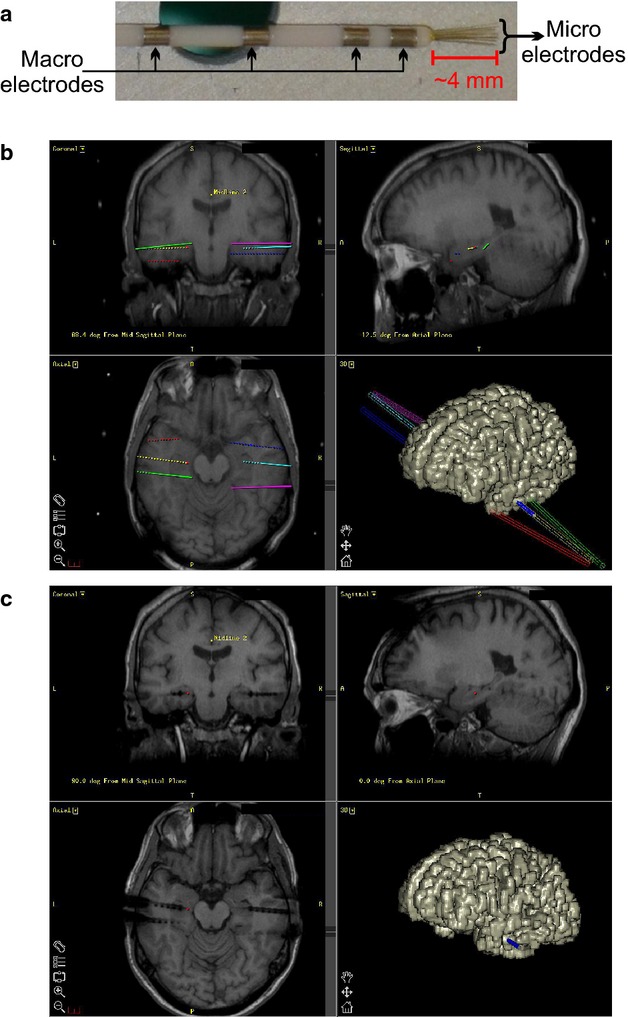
Surgical planning. (A) Before implantation, the microwires are inserted in the lumen of the polyurethane probe so they can be trimmed to the desired length (∼4 mm). (B) Based on a pre-operative MRI scan, computer software provides the stereotactic coordinates of the targets where the electrodes should be implanted. (C) After surgery, a post-operative MRI or CT is co-registered with the preoperative MRI to assess the accuracy of the actual position of the electrodes.
